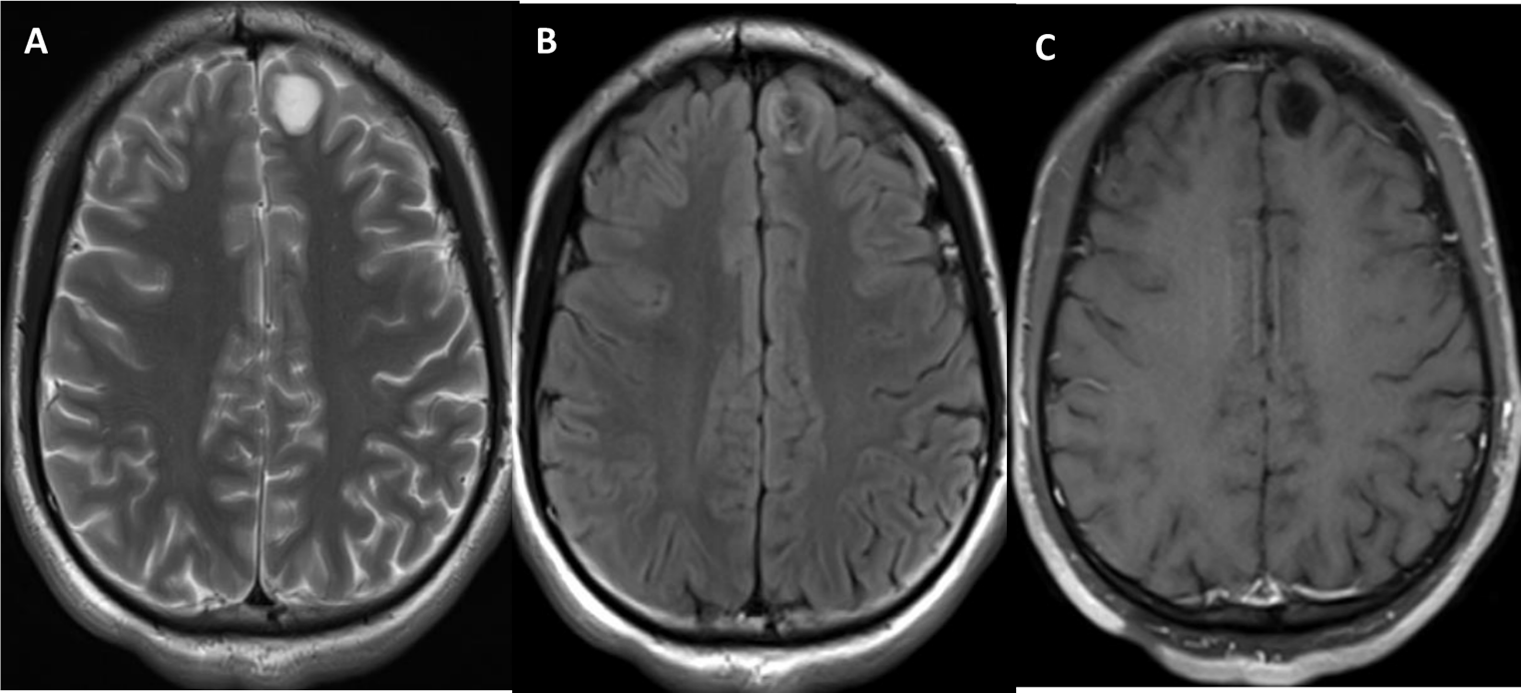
Pure cystic PGNT in the left frontal lobe of a 19-year-old male. (A) The lesion on axial T2WI shows uniform hyperintensity. (B) Axial T2-FLAIR shows “T2 FLAIR mismatch” sign. (C) Axial enhancement shows no enhancement of the lesion.
